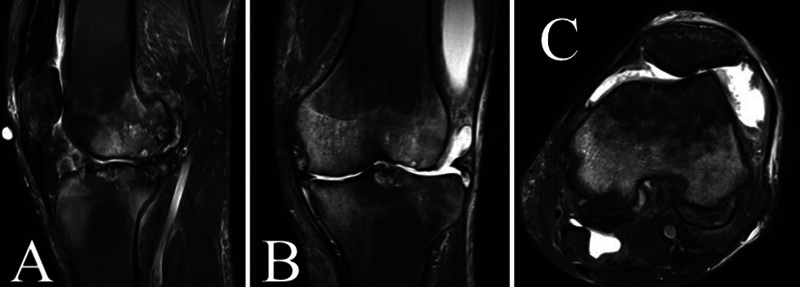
Magnetic resonance imaging of the left knee. (
